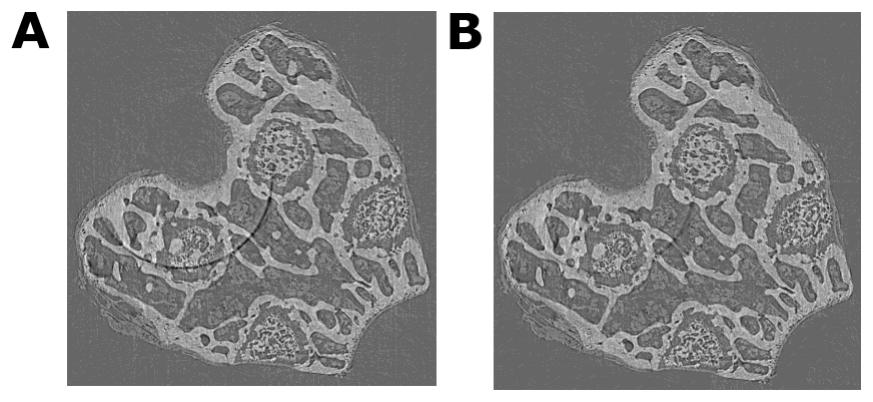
Before and after CT image via image preprocessing. (A) 2D CT image without image preprocessing. (B) 2D CT image after image preprocessing.
